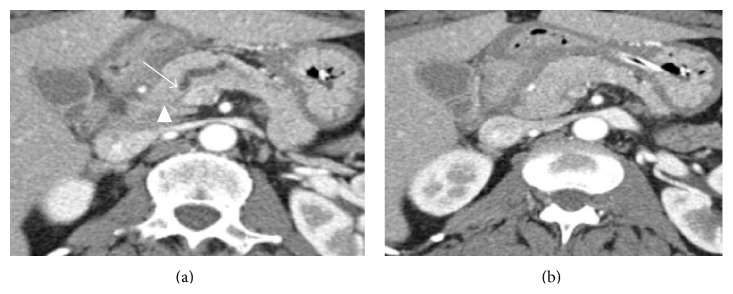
Contrast-enhanced computed tomography images obtained during the pancreatic phase. (a) Axial CT image of the pancreatic head shows abrupt termination of a markedly dilated pancreatic duct (arrow) due to a surgically proven pancreatic cancer causing duct obstruction. No mass is visualized in the region of the duct-obstructing tumor (arrowhead). (b) Image of the pancreatic head 1 cm inferior to image (a) shows homogeneous enhancement without evidence of hypoattenuation changes or mass effect despite the presence of large (3.9 cm) diffusely infiltrating pancreatic ductal adenocarcinoma tumor.
